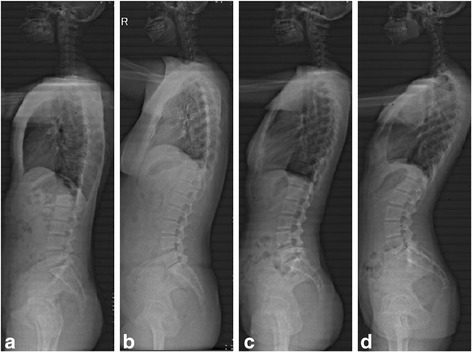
Panoramic radiographic of four types of sagittal alignment. a Roussouly type I. b Roussouly type II. c Roussouly type III. d Roussouly type IV
